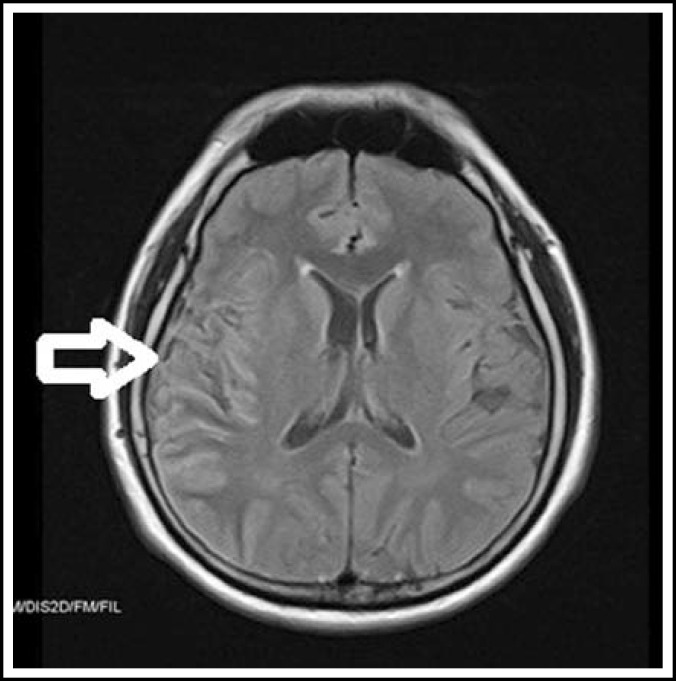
MRI brain before treatment showing bright T2/FLAIR cortical images at the right parietal and temporal region
